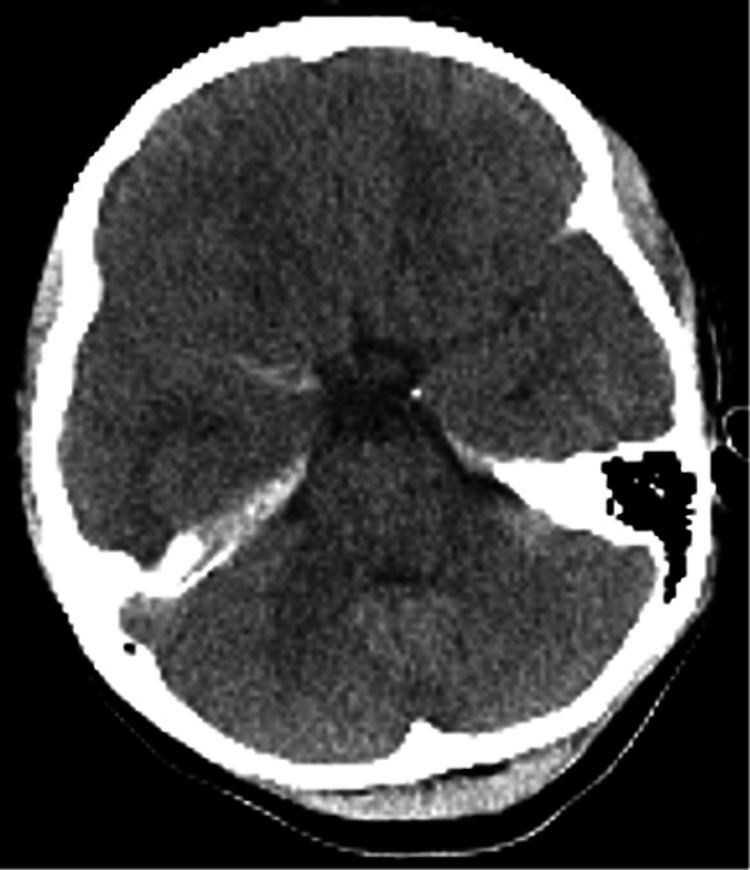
A CT scan of the head on the first day revealed a right temporal lobe cerebral contusion, an acute subdural hematoma in the tentorium, and a traumatic subarachnoid hemorrhage in the Sylvian fissure. No intracerebral hematoma and brain edema were observed. CT: computed tomography
